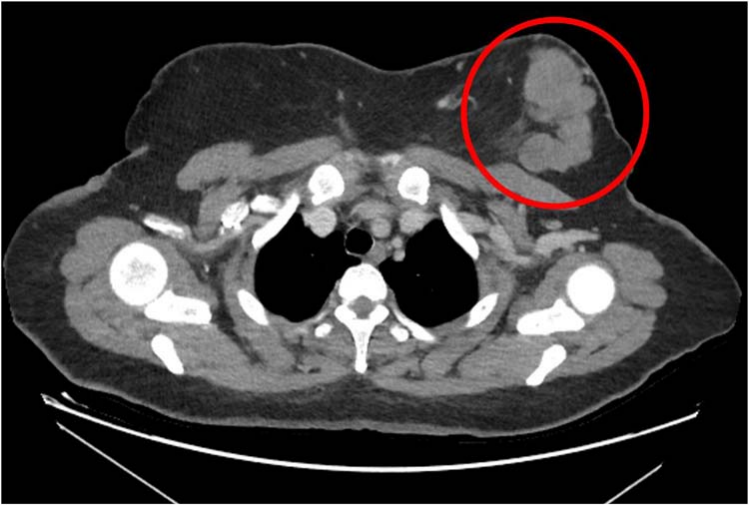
Breast mass seen on baseline CT scan, encircled.
